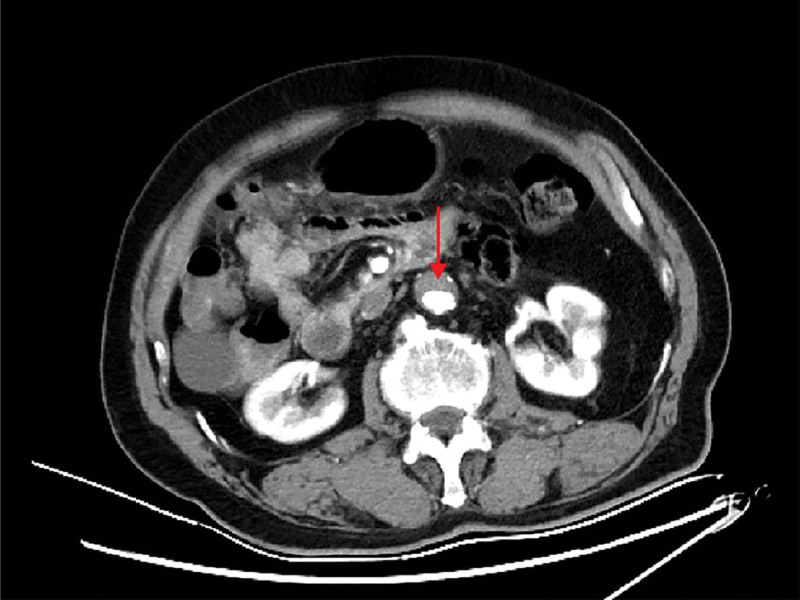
Computed tomography angiography of the chest and abdomen revealed atherosclerosis of the abdominal aorta with thrombus formation.
